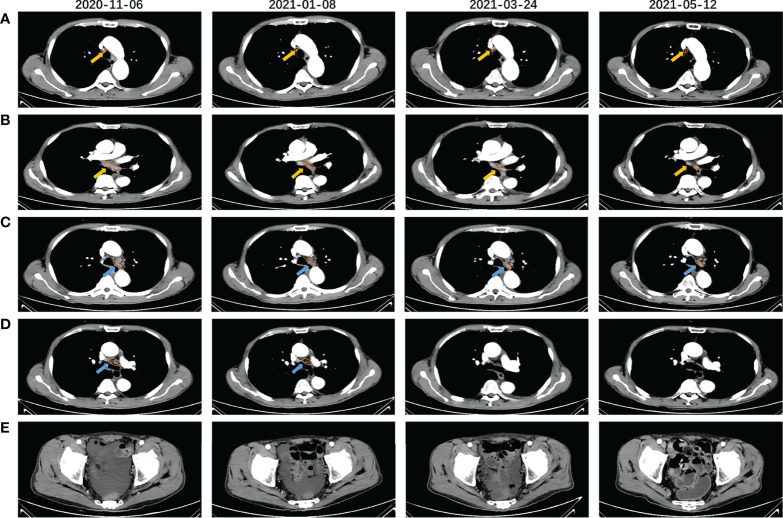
CT scans before (2020-11.06), during (2021-01-08), and after (2121-03-24, 2021-05-12) the PRaG therapy. Right upper paratracheal (A) and subcarinal lymph node (B) were the two irradiated lesions that gradually shrunk significantly after the PRaG therapy, One of the left lower paratracheal lymph nodes (C) progressively shrunk and the other (D) rapidly shrunk and disappeared after PRaG therapy. (E) shows that pelvic effusion gradually decreased and disappeared, The yellow arrow indicated the irradiated lesions, and the blue arrow indicated the unirradiated ones; all assessed lesions were circled with orange lines.
